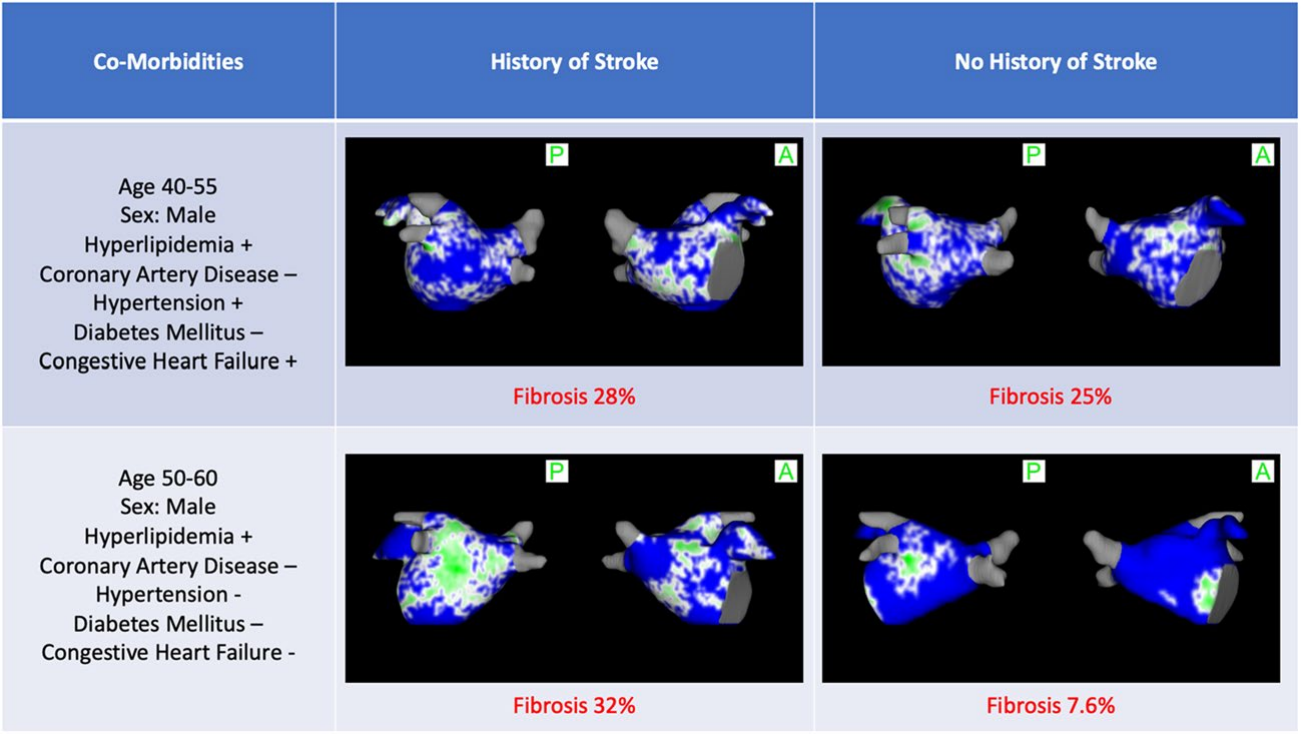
Baseline LGE-MRI scans of two patients with a history of stroke and two patients without a history of stroke with similar comorbidities
